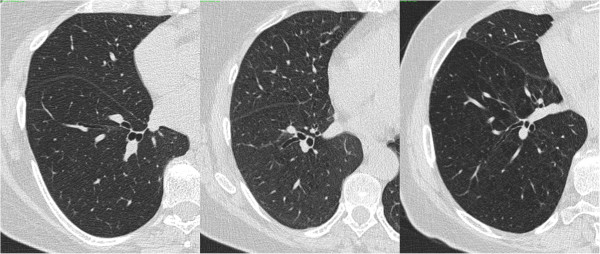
Axial inspiratory CT scans at the level of right inferior pulmonary vein, from three 54 year old females, representing typical patterns; Left panel: healthy never-smoker; Middle panel: smoker (note subtle uniform diffuse increased opacity which makes vessels more visible and mild interlobular septal thickening, especially in middle lobe; Right panel: COPD (note diffusely distributed centrilobular emphysema, more in central part of scans, with radiolucency and irregular vascular pattern).
